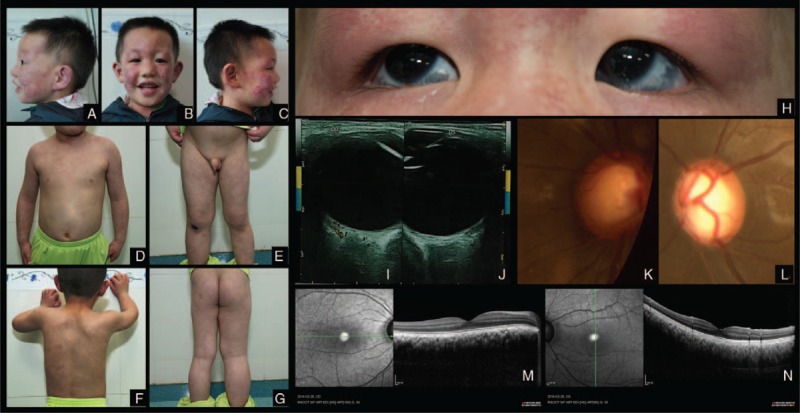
Case 3. Reticular, marble-like vascular lesions (CMTC) on the face along the branches of trigeminal nerve. Bilateral triangular alopecia on temporal scalp (A–C). CMTC on the neck, chest, arms and hands, aberrant blue spots on the chest and abdomen (D). CMTC and aberrant blue spots on the back (F). CMTC on the legs. Hemihypertropy on the right lower extrimety (E, G). Scleral hyperpigmentation in both eyes (H). Increased choroidal thickness and increased internal blood flow in the lesion on color Doppler ultrasonography in both eyes (I, J). Enlarged optic cup in both eyes (K,L). Increased choroidal thickness in the right eye on OCT scan (M). Increased choroidal thickness in the left eye on OCT scan (N).
